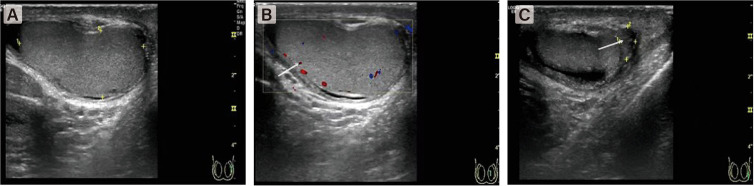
Color Doppler US image shows a few of color flow signal at the intratesticular tissue. (A) The right testicle was enlarged and axial position changed. (B) The blood flow signal in the testis was significantly reduced. (C) The boundary between epididymis and testis is not clear.
